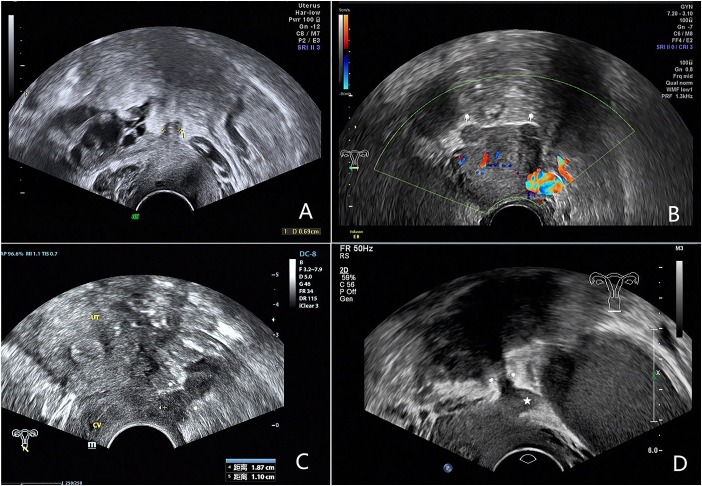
Images showing four types of USL lesions according to ultrasound characteristics of USL endometriosis. (A) Type I: the root segament of USL is thickened and stiff. (B) Type II: the arrows show endometriosis nodules on both sides of the USL. (C) Type III: the arrows show hypoechogenic irregular striped endometriosis lesion. (D) Type IV: the arrows show irregular striped endometriosis lesion of the right USL, the Pentagram shows a nodule of the left USL.
